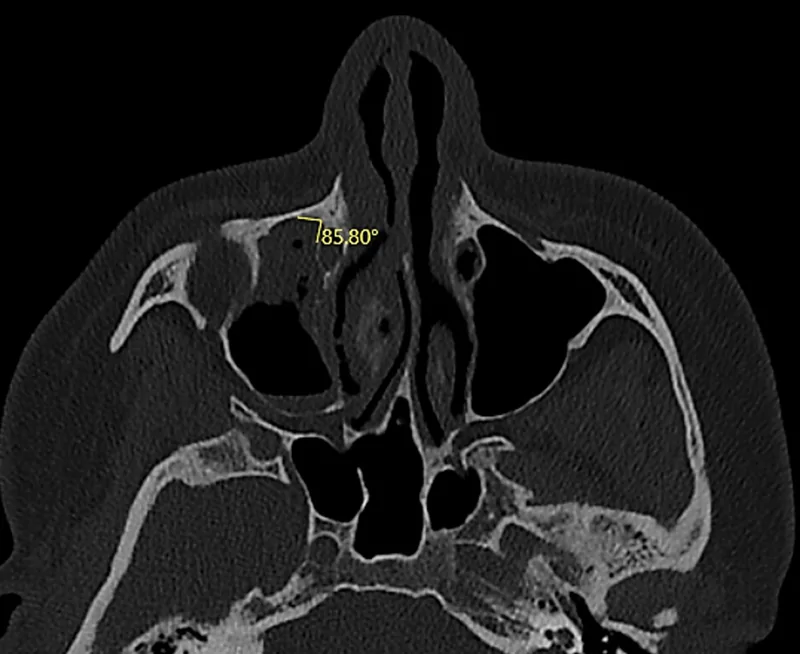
Computed tomography scan showing low grade feasibility of the TPRA in right CRS type Ib, with the yellow line representing the angle of the TPRA.
